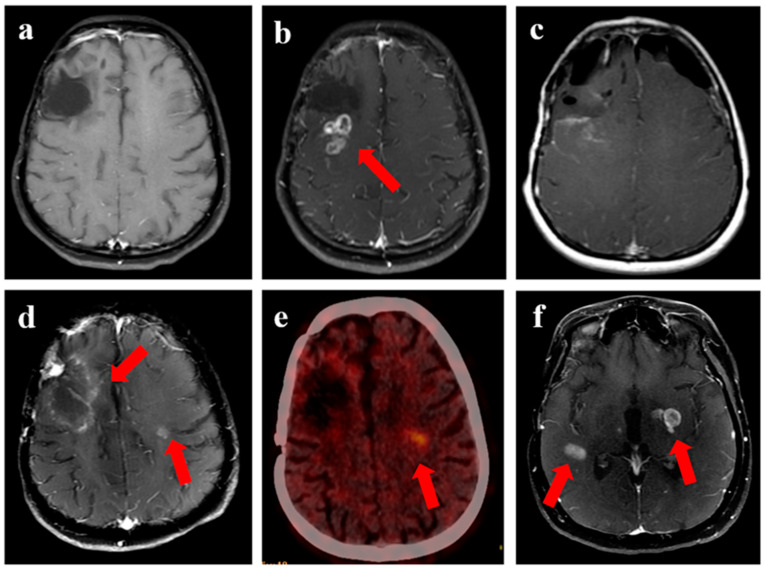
Disease evolution from first progression to subsequent recurrence following re-irradiation in a 25-year female with IDH-mutant grade 3 astrocytoma. (a) shows T1w contrast-enhanced MRI with resection cavity over the right frontal region, with contrast-enhancing recurrent disease seen over the posterior aspect of the cavity (arrow) in (b). Subsequent imaging shows MRI following resection of the disease in (c), which was confirmed as recurrent HGG. (d) shows an enhancing area with central necrosis over the resected and re-irradiated region (8 months following ReRT) over the right frontal-parietal, which was not showing any metabolic uptake on corresponding PET imaging in (e). However, the other area of enhancing nodule over the left periventricular region shows high avidity, considered a distant recurrence. (f) shows MRI performed after 2 months, which confirmed new areas of distant disease, while the re-irradiated region (non-avid on PET) continued to be stable, confirming RT-related changes.
